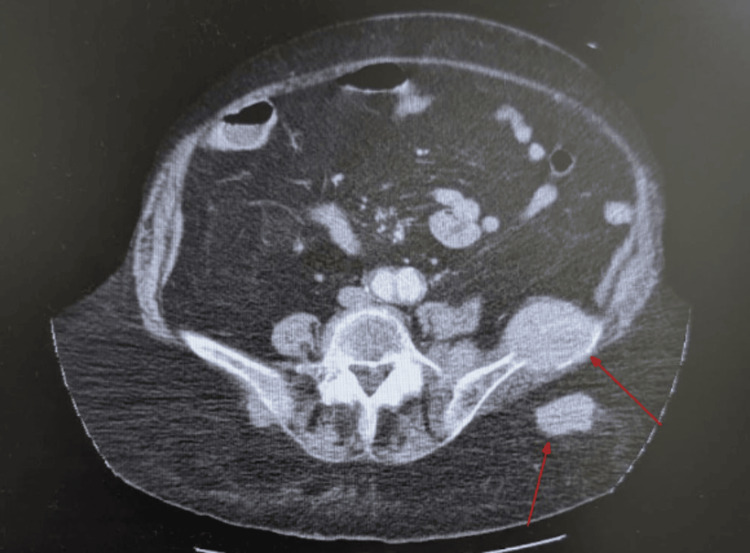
CT with IV contrast depicting bony and buttock metastatic lesions Soft tissue mass at the left iliac wing measuring 4.6 x 5.4 cm with erosive changes and a left buttock mass measuring 2.4 x 4.0 cm. Interventional radiology-guided biopsy was performed on the iliac lesion, and pathology confirmed the diagnosis of a high-grade neuroendocrine carcinoma.
